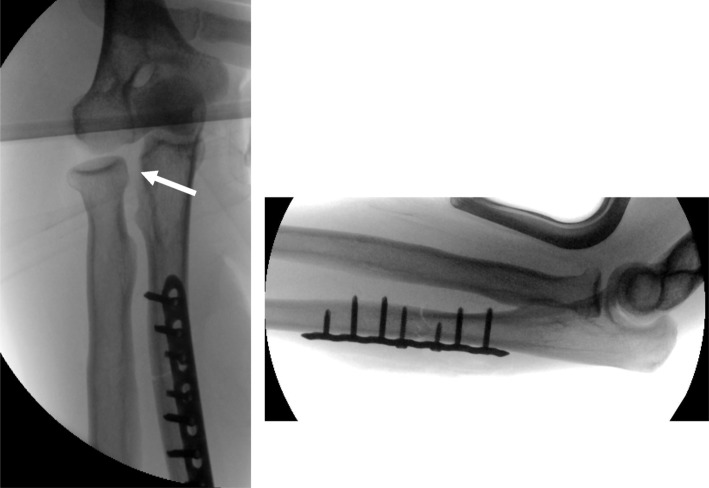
Intraoperative X-ray after plate osteosynthesis of a Monteggia fracture with remaining instability in the PRUJ (white arrow)
